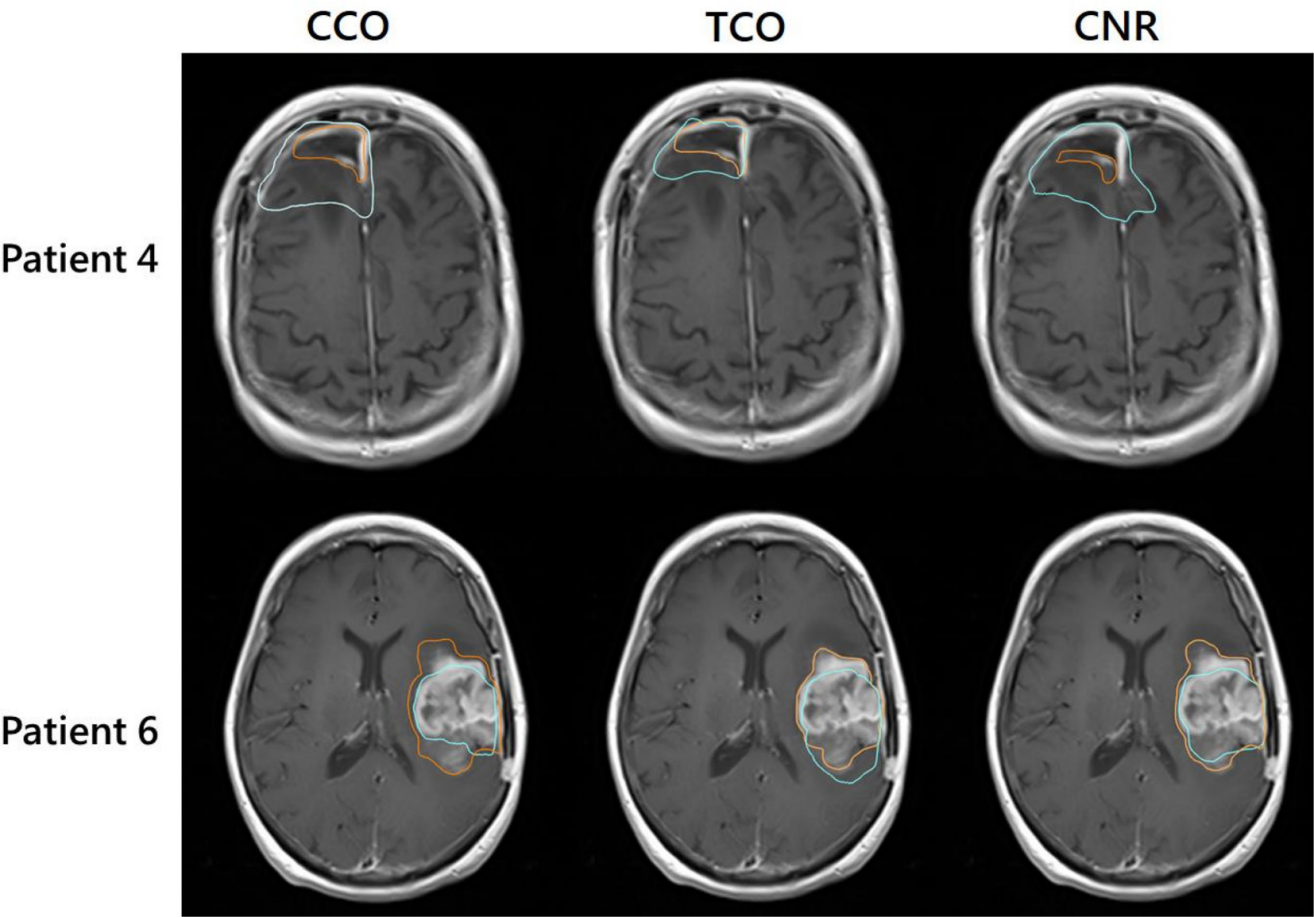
Axial slices of pre-RT MRIs for patients 4 and 6, with pre-RT (orange) and post-surgical (blue) delineations. Patient four showed the largest difference in GTVs, with post-surgical GTVs being larger than pre-RT for all observers. Patient six was the only patient that showed signs of progression between MRI acquisitions, and post-surgical GTV delineations were smaller than pre-RT GTVs for: consultant clinical oncologist (CCO), trainee clinical oncologist (TCO), and consultant Neuroradiologist (CNR)
